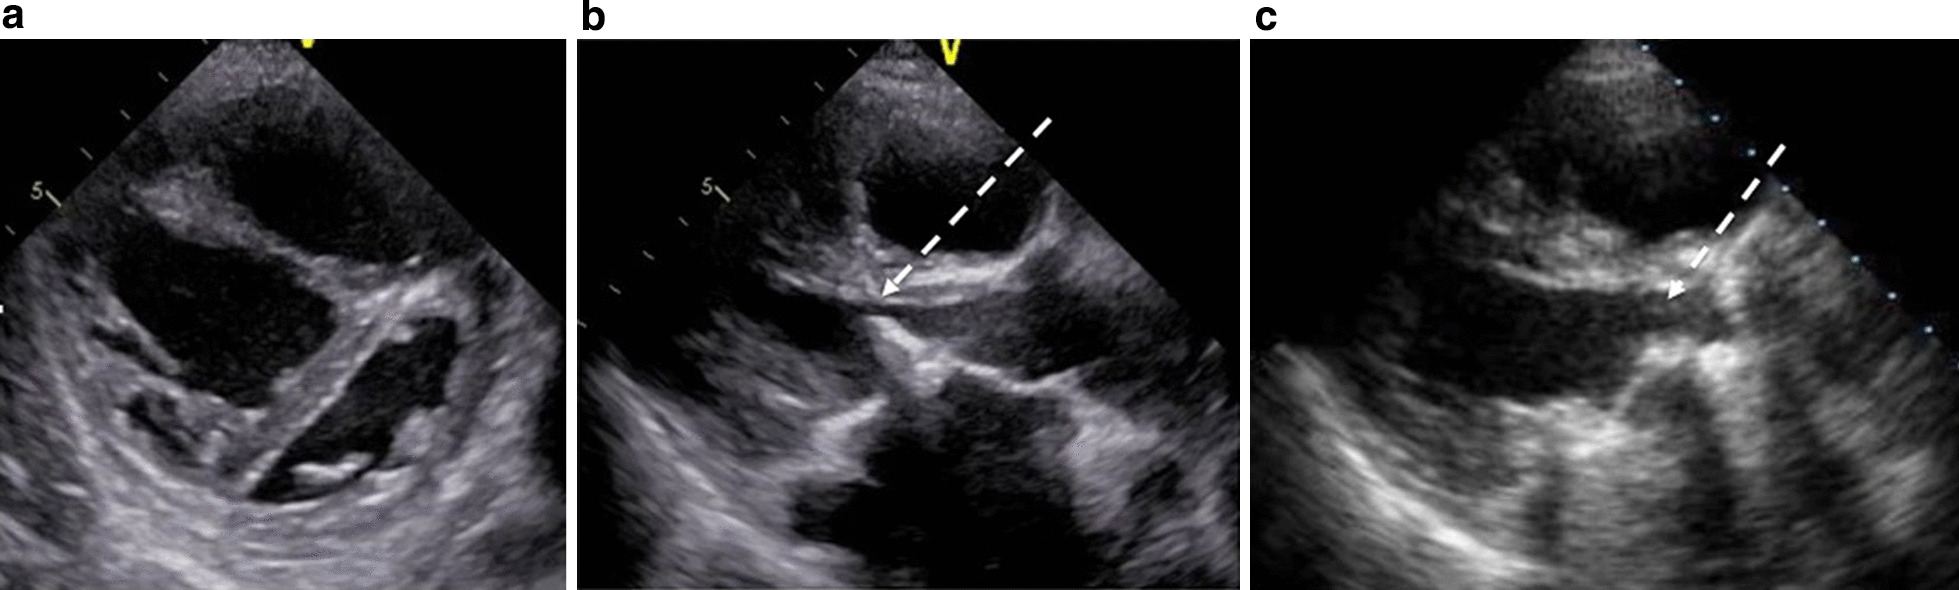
a Preoperative echocardiography shows a small left ventricle being compressed by a dilated right ventricle. b Preoperative echocardiography shows left ventricular outflow tract obstruction due to stent-post protrusion of the bioprosthetic mitral valve (dotted arrow). c Postoperative echocardiography shows improvement of left ventricular outflow tract obstruction (dotted arrow)
